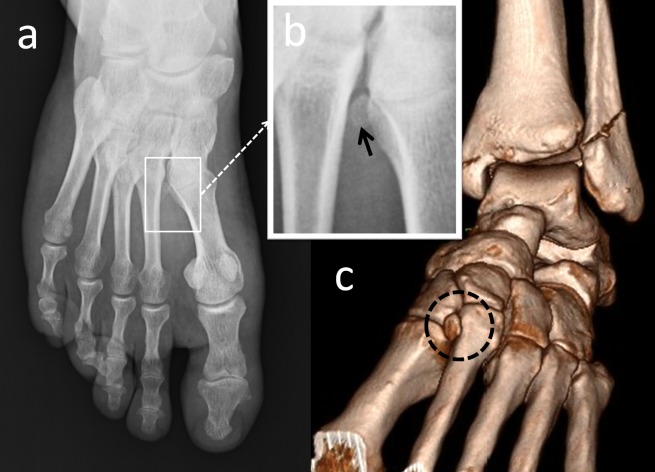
An os intermetatarseum (a) Anteroposterior foot radiograph; (b) Magnified view of the intermetatarsal space, the black arrow is os intermetatarseum; (c) 3D computed tomography (CT) appearance.
